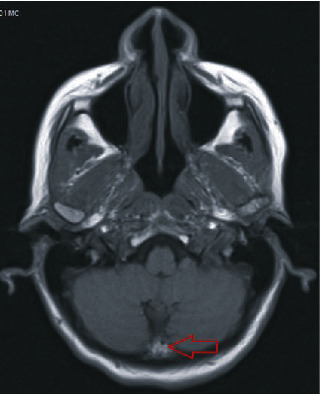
Patient MRI in axial view. Demonstrates calcifications in the supratentorial cerebellum.
